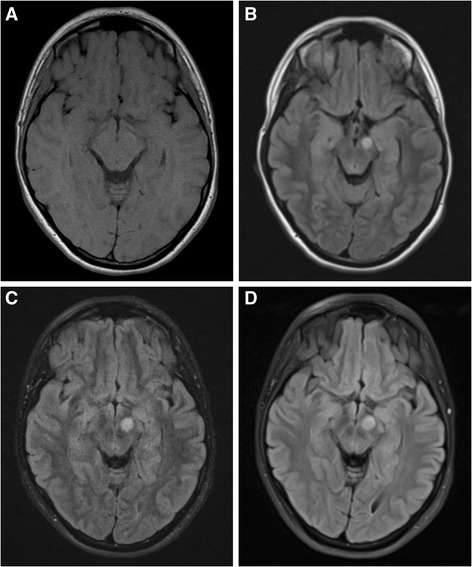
Newly-appearing glioma in the left cerebral peduncle in Patient 19. a There is no visible glioma on the patient’s first scan. b 4 years later, an enhancing glioma has appeared in the left cerebral peduncle measuring 0.5 cm^3^. c 5 years after the initial glioma-free scan, the glioma has increased to a volume of 0.8 cm^3^. d 7 years after the initial scan, the glioma measured 1.3 cm^3^. All images shown are FLAIR sequences. The patient remained asymptomatic during follow-up and also has an optic glioma (not visible in these images)
